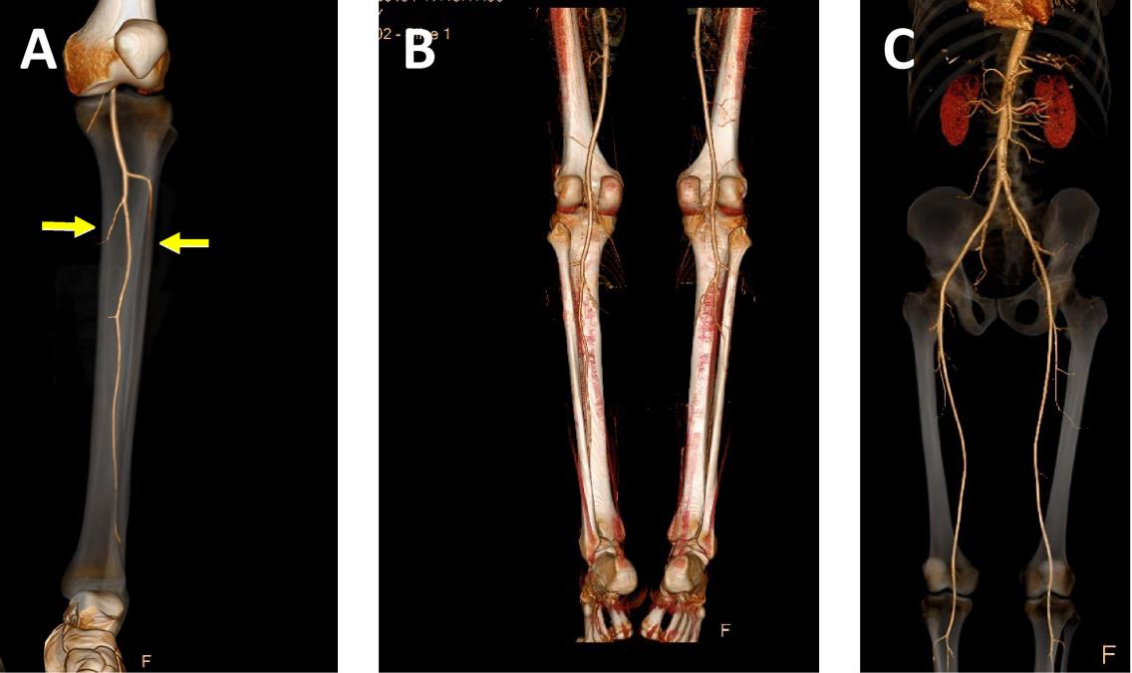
Computed Tomography Angiography of Thromboangiitis Obliterans (TAO)–like Vasculopathy. The patient’s CTA revealed severe vasculopathy of the lower legs with bilateral arterial occlusion of the anterior and posterior tibialis (arrows), without distal reperfusion (A). There were no signs of atherosclerosis, and the proximal vessels were normal (B, C). These imaging findings resembled TAO. Image quality enhanced for publication using AI Image Upscaler (Zyro.com).
